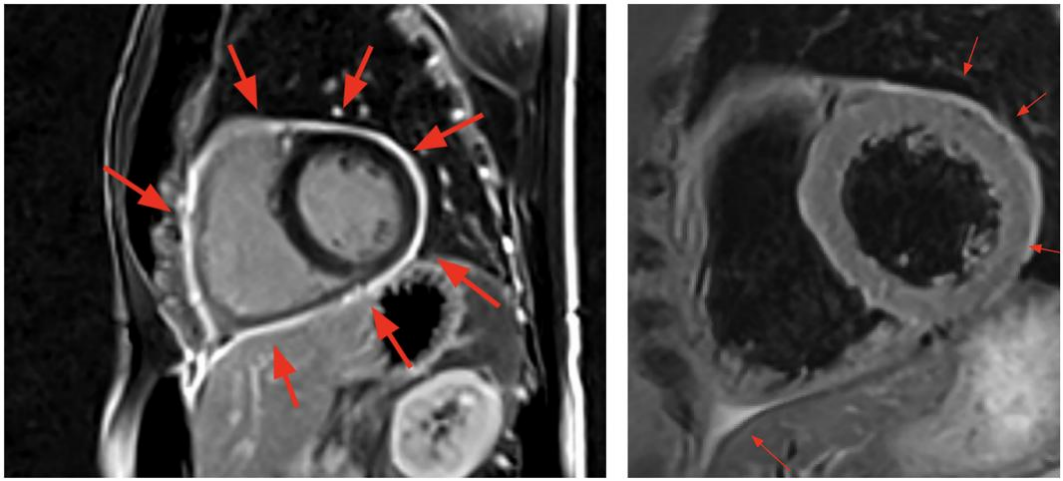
Cardiac MRI with late gadolinium enhancement and T2 Stir. The left panel demonstrates a sagittal late gadolinium enhancement image, highlighting circumferential pericardial delayed enhancement (red arrows), consistent with pericardial inflammation and scar. The right panel displays a T2 STIR image, which reveals pericardial oedema (red arrows).
